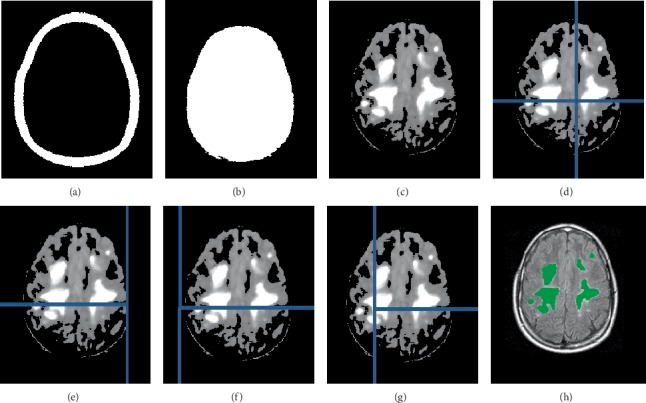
Morphological operations on MRI image and detection of tumors, (a) The largest surrounding edge expanded inward, (b) the largest remaining connected component, (c) The modified MRI image, (d) splitting the MRI image into 200 × 200 patches, (e–g) Shifting the horizontal indices before repeating the detection process (h) The resultant detected tumors.
